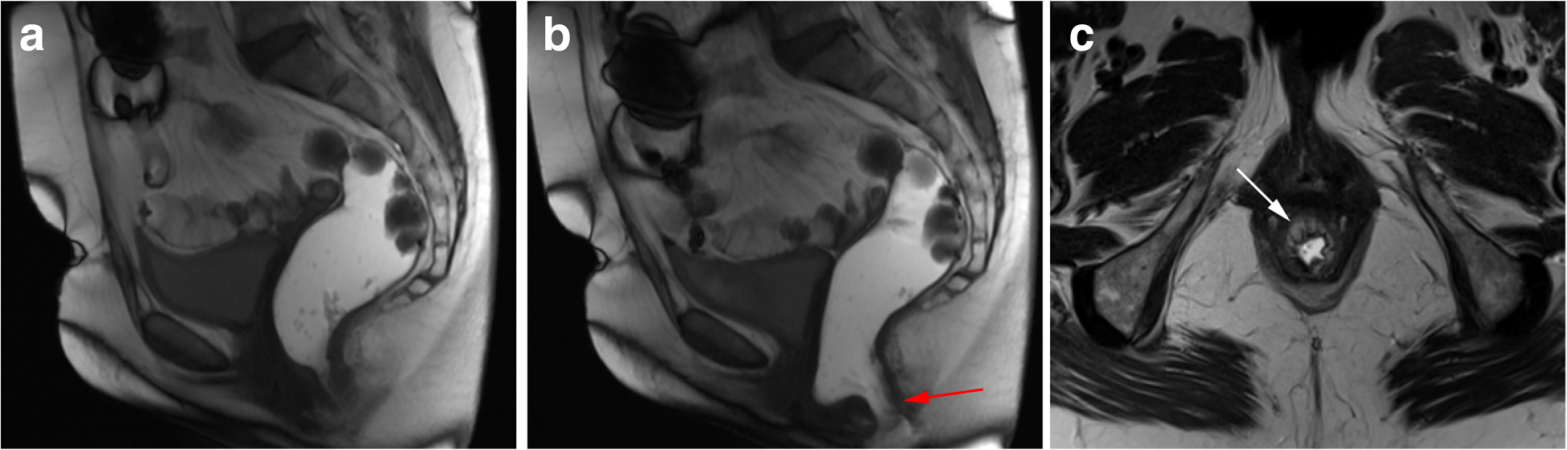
Anal incontinence. (a, b) Sagittal midline TrueFISP images at rest (a) and during Valsava maneuver (b), with loss of the ultrasound gel in the second one. Notice the opening of the anal sphincter (red arrow). (c) Axial T2-TSE image of the internal anal sphincter demonstrating atrophy with lipomatous involution of the sphincter muscles (white arrow)
